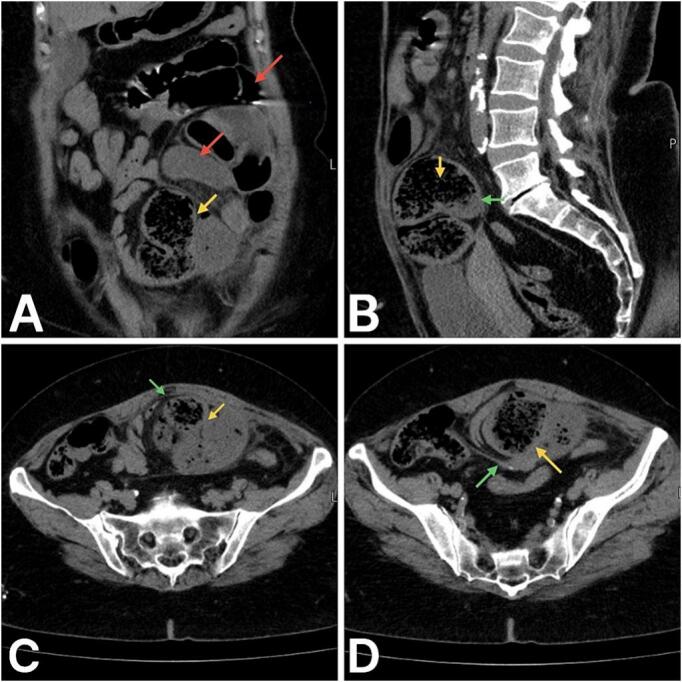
Abdominal CT without IV contrast. A: Coronal image shows proximal dilated small bowel loops (red arrows) and the encapsulated C-shaped configuration of the closed-loop (yellow arrows). B, C, and D are sagittal, sagittal and axial sections, respectively, which show a thin layer of the peritoneum (green arrow) surrounding the closed loop (yellow arrow). (For interpretation of the references to colour in this figure legend, the reader is referred to the web version of this article.)
